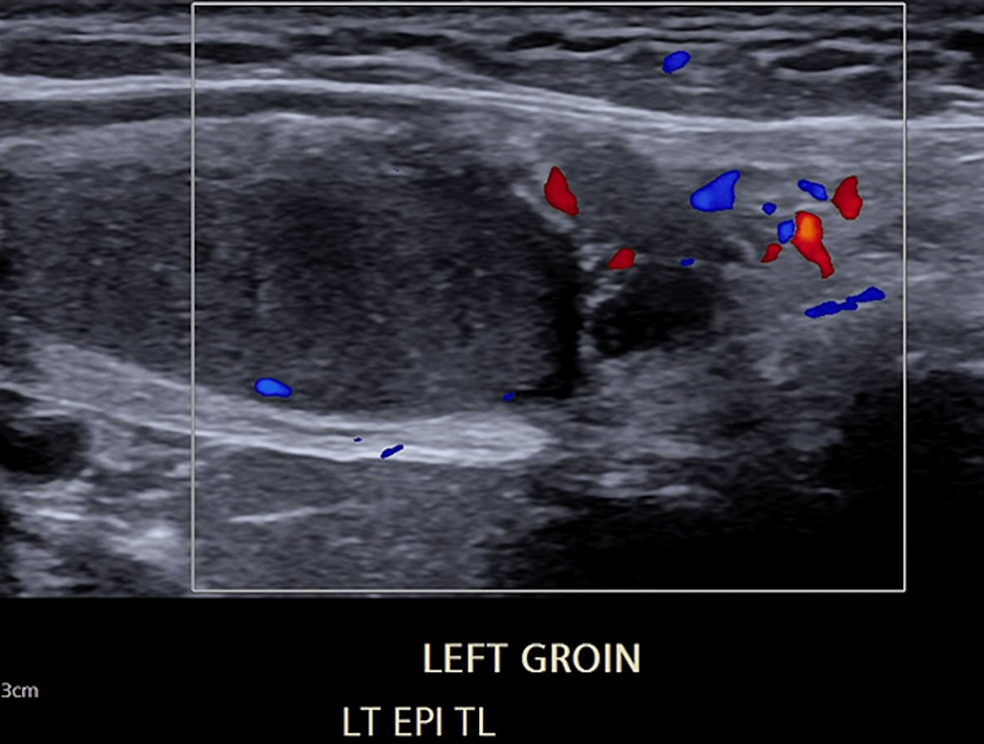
Pre-operative ultrasound of the patient in case 2 Ultrasound of the patient in case 2 a year ago, which showed normal size (4.5 x 2.7 x 1.7 cm), shape, and echotexture of the right testicle, with scattered microliths; the left testicle was in the left groin, smaller in size (3.6 x 2.0 x 1.5 cm), and normal in shape and echotexture, with no focal lesions.
